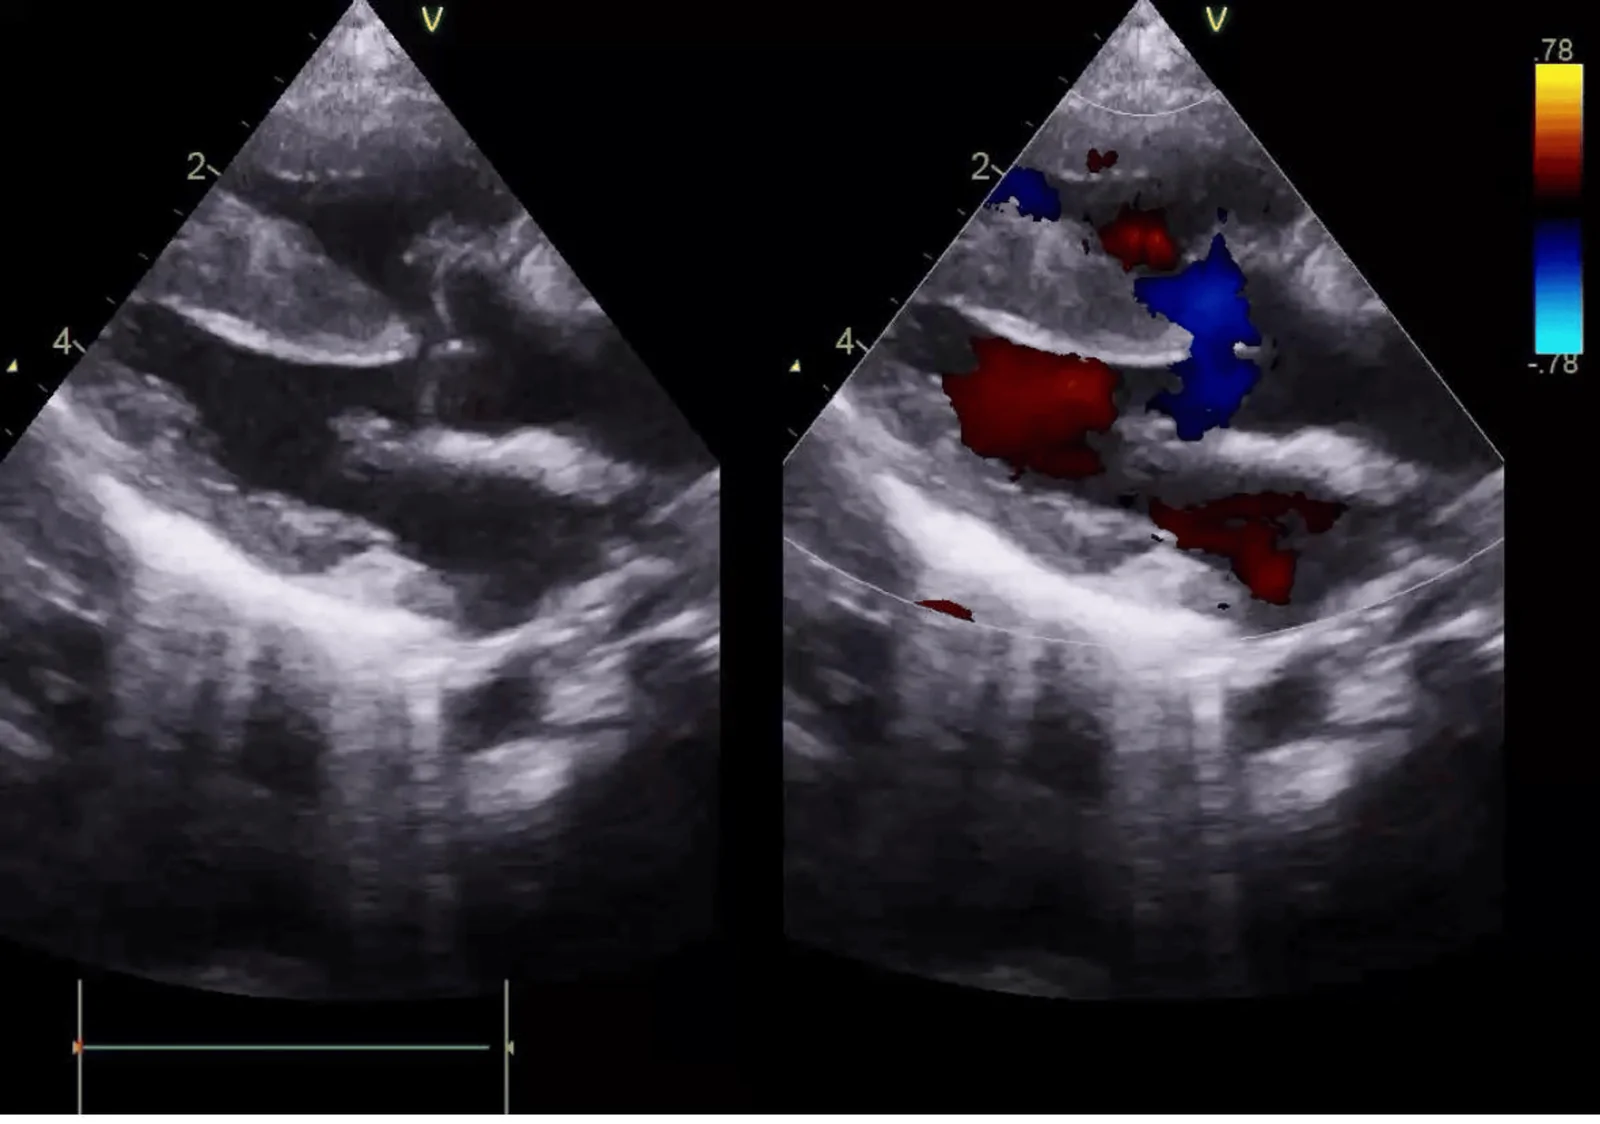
ECHO showing the parasternal long-axis view with a VSD and overriding of the aorta ECHO: echocardiogram; VSD: ventricular septal defect
